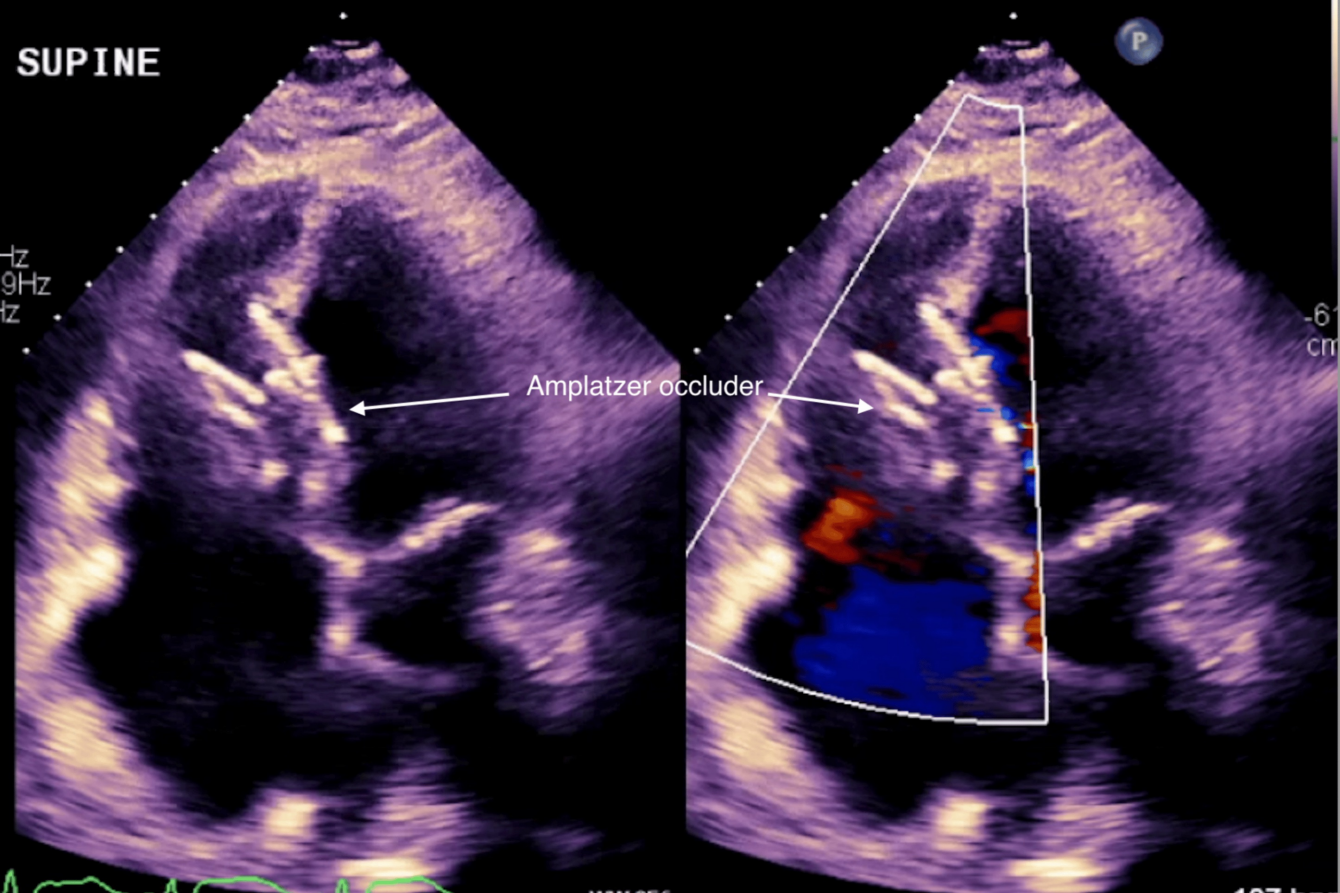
TTE with color flow post VSD closure with Amplatzer occluder TTE: transthoracic echocardiography, VSD: ventricular septal defect
